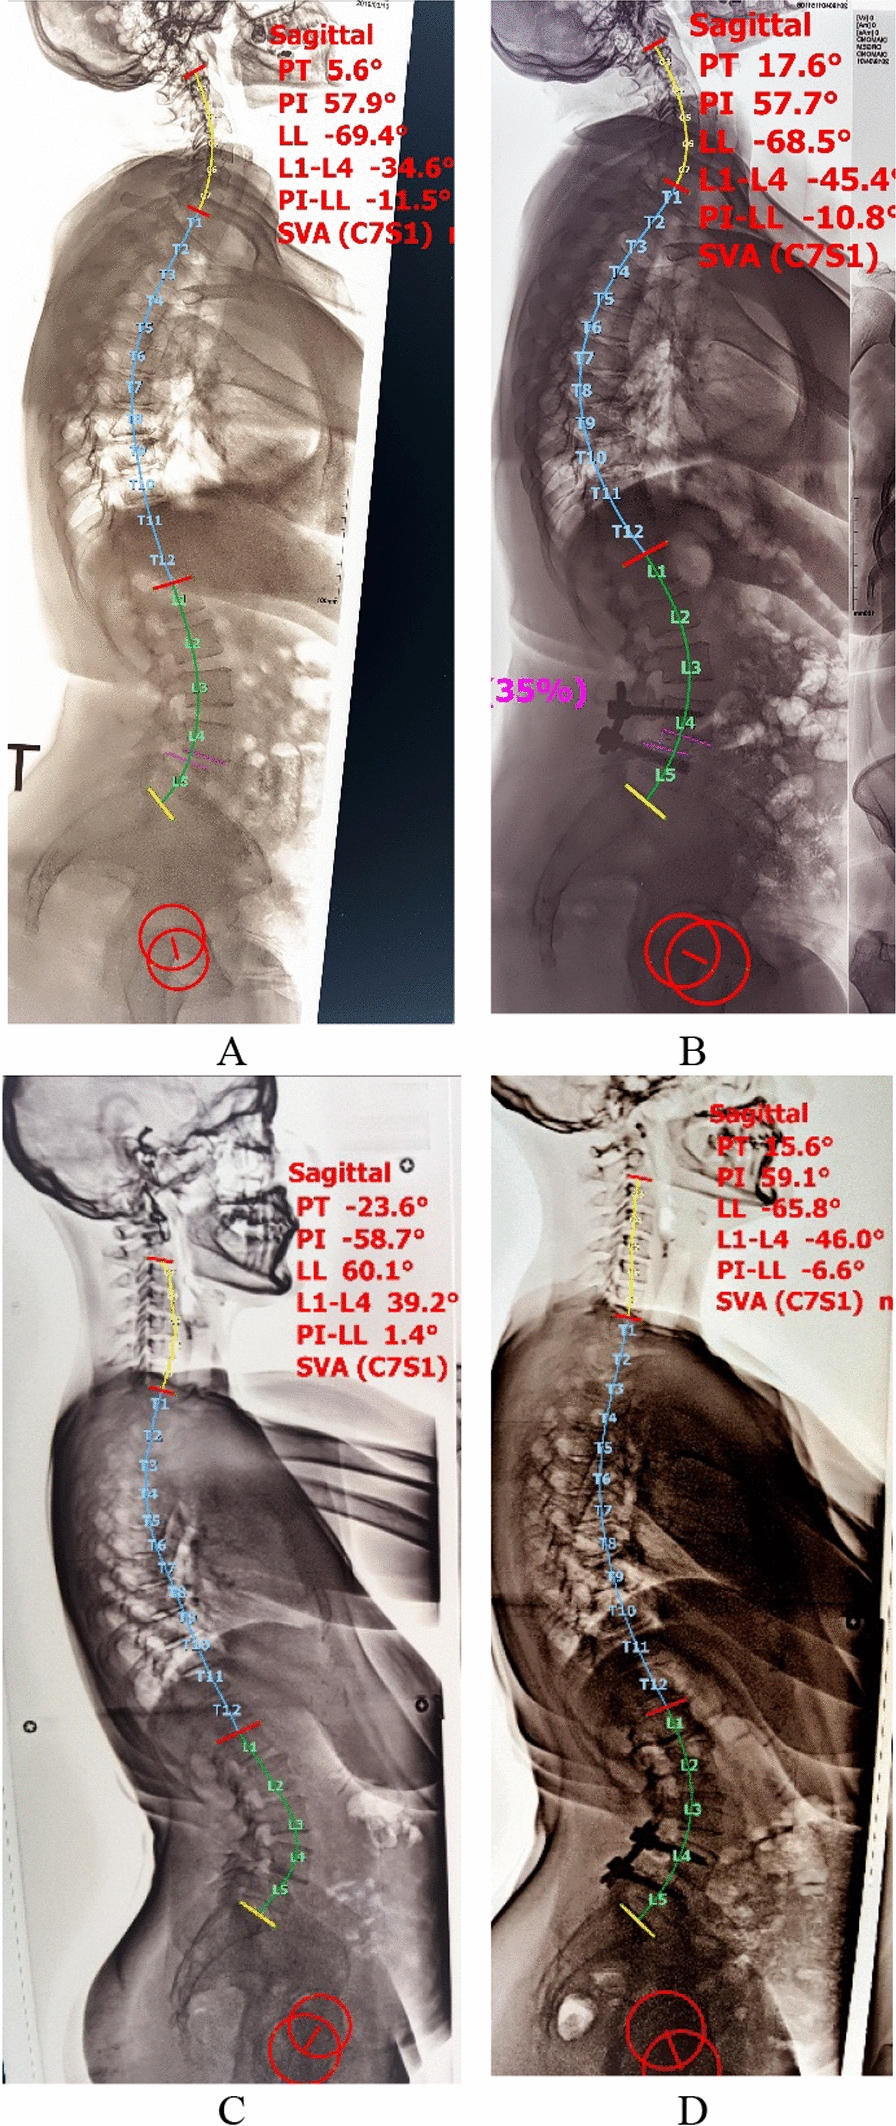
Long standing radiographyof the whole spine pre and postoperative of the open-TLIF case in (A and B) and the MIS-TLIF case (C and D) with measurement of the spinopelvic parameters
